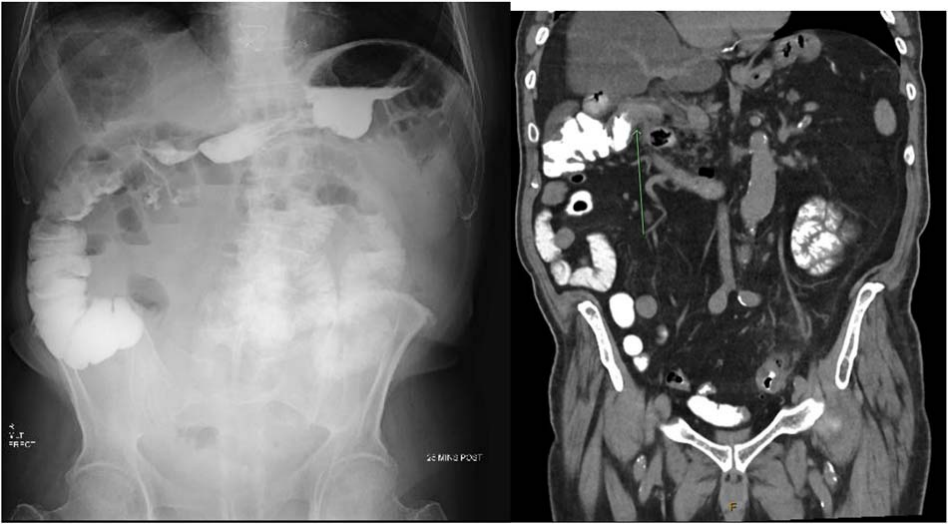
X-ray post barium swallow demonstrating early contrast opacification of the ascending colon with coronal slice of CT confirming the presence of a fistula between the duodenum and ascending colon below the hepatic flexure.
